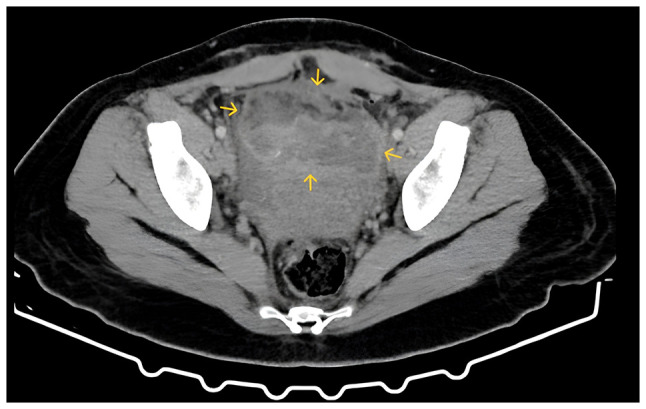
Computed tomography scan revealing a pelvic abscess at the uterine scar at the level of the isthmus (yellow arrows), characterized by a large, irregular, inhomogeneous solid-cystic mass with entrapped intralesional air bubbles.
